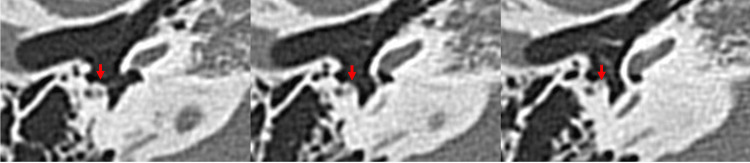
Axial HRCT images of the right temporal bone (Case 1) showing a hypoplastic pyramidal eminence (arrows). Three consecutive sections shown are 0.5 mm thick. HRCT, High-resolution computed tomography
